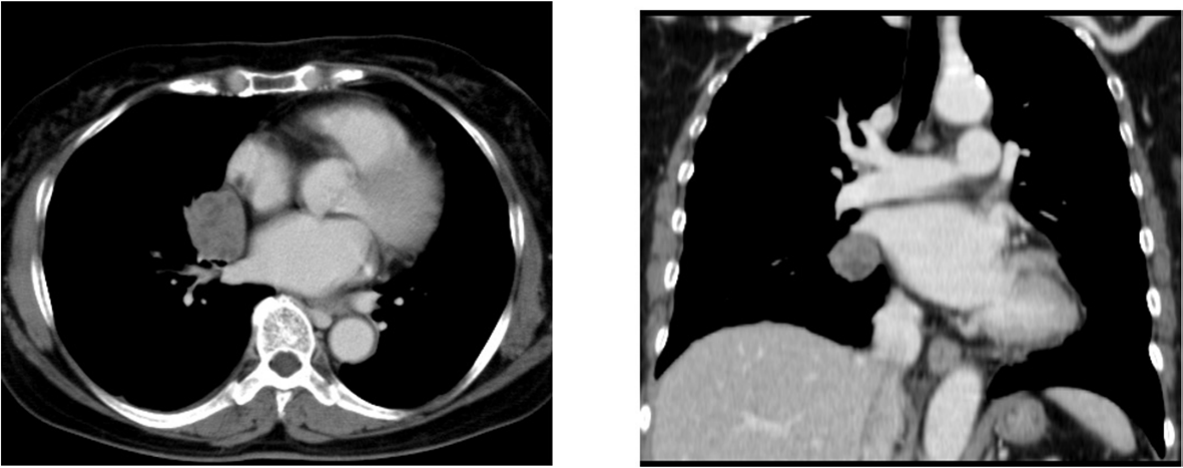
Chest computed tomography images. A well-defined lesion in the right hilum of the lung
